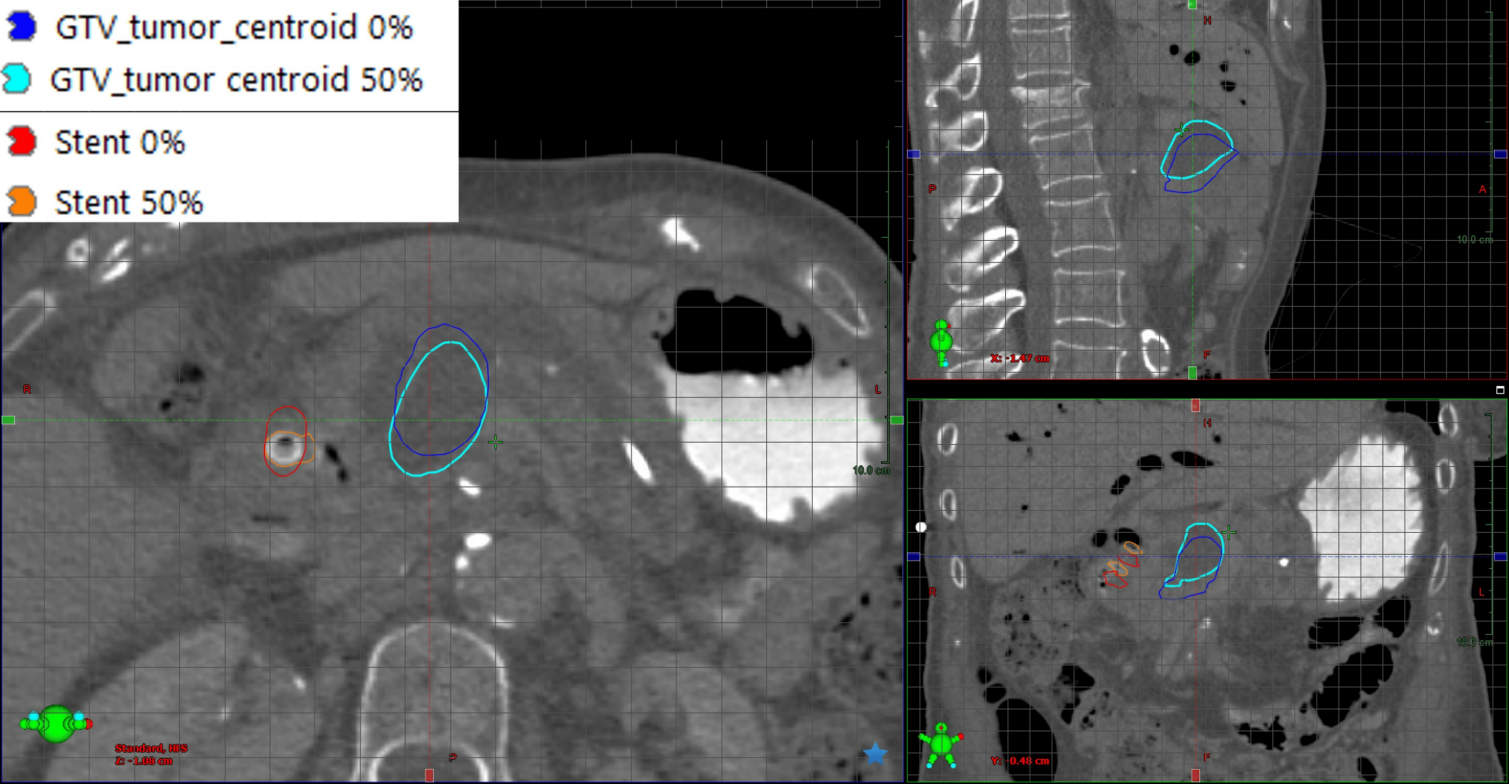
50% phase CT image showing the extrema GTV and stent positions Grid lines show a 1 cm spacing GTV: Gross Tumor Volume
